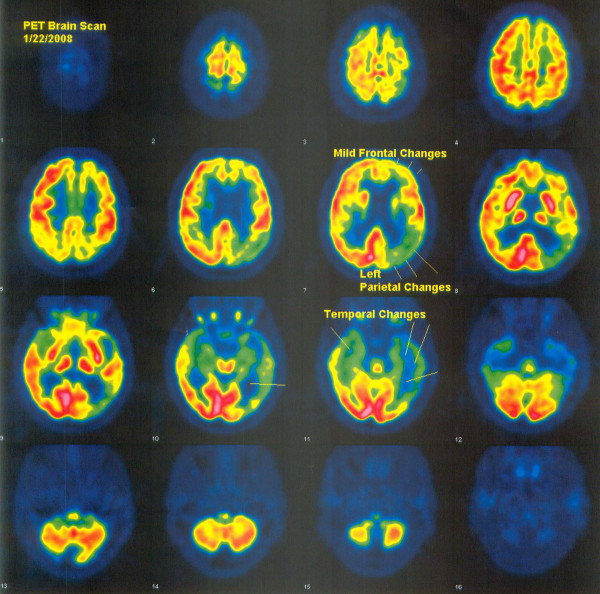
FDG-PET brain scan of a patient with non-fluent aphasia secondary to Alzheimer's disease (case report 2), prior to treatment, demonstrating decreased glucose metabolism in the left parietal, bilateral temporal, and left frontal lobe.
